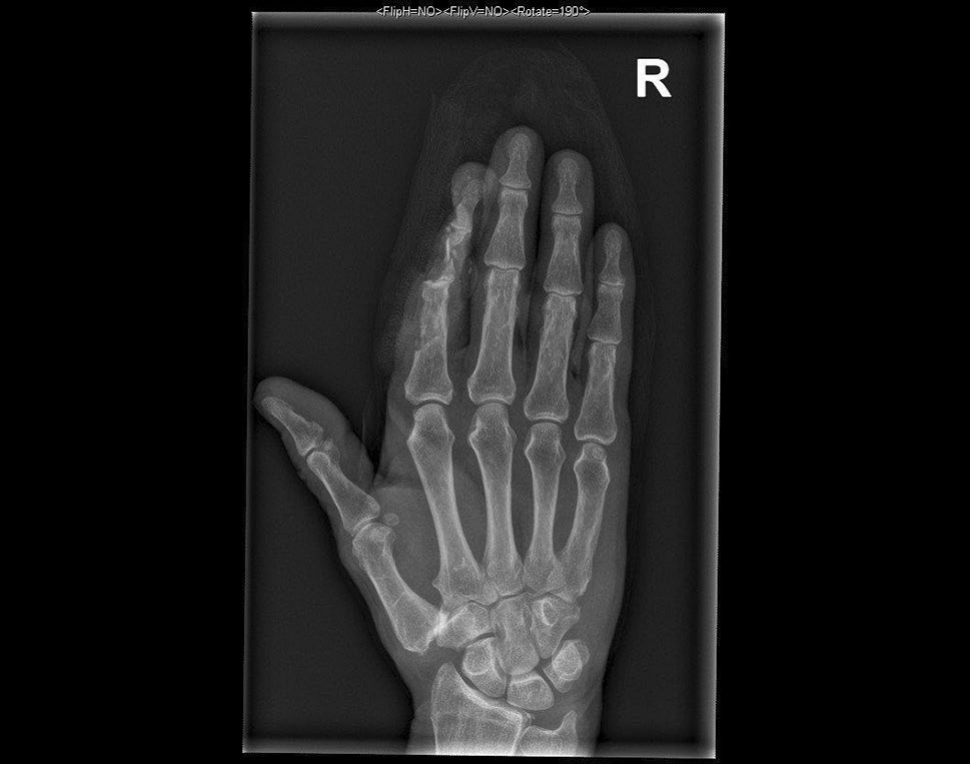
Case Presentation 3: X-ray after circular saw injury with loss of a major part of P2 and P3 of the index finger
